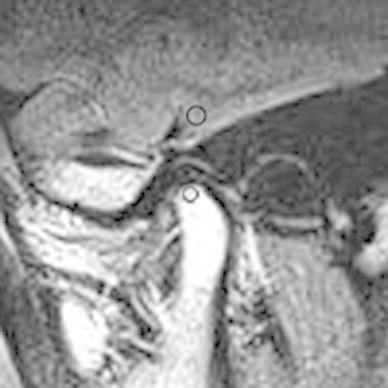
Measurement of region of interest (ROI) on corrected sagittal FLAIR images in closed-mouth position. The *lower circle* represents a 4.5-mm^2^ ROI in the bone marrow of the mandibular condyle; the *upper circle* represents a 5.9-mm^2^ ROI in the gray matter. Both were placed as close as possible to the *top* of the condyle on a *line* running perpendicular through its length
